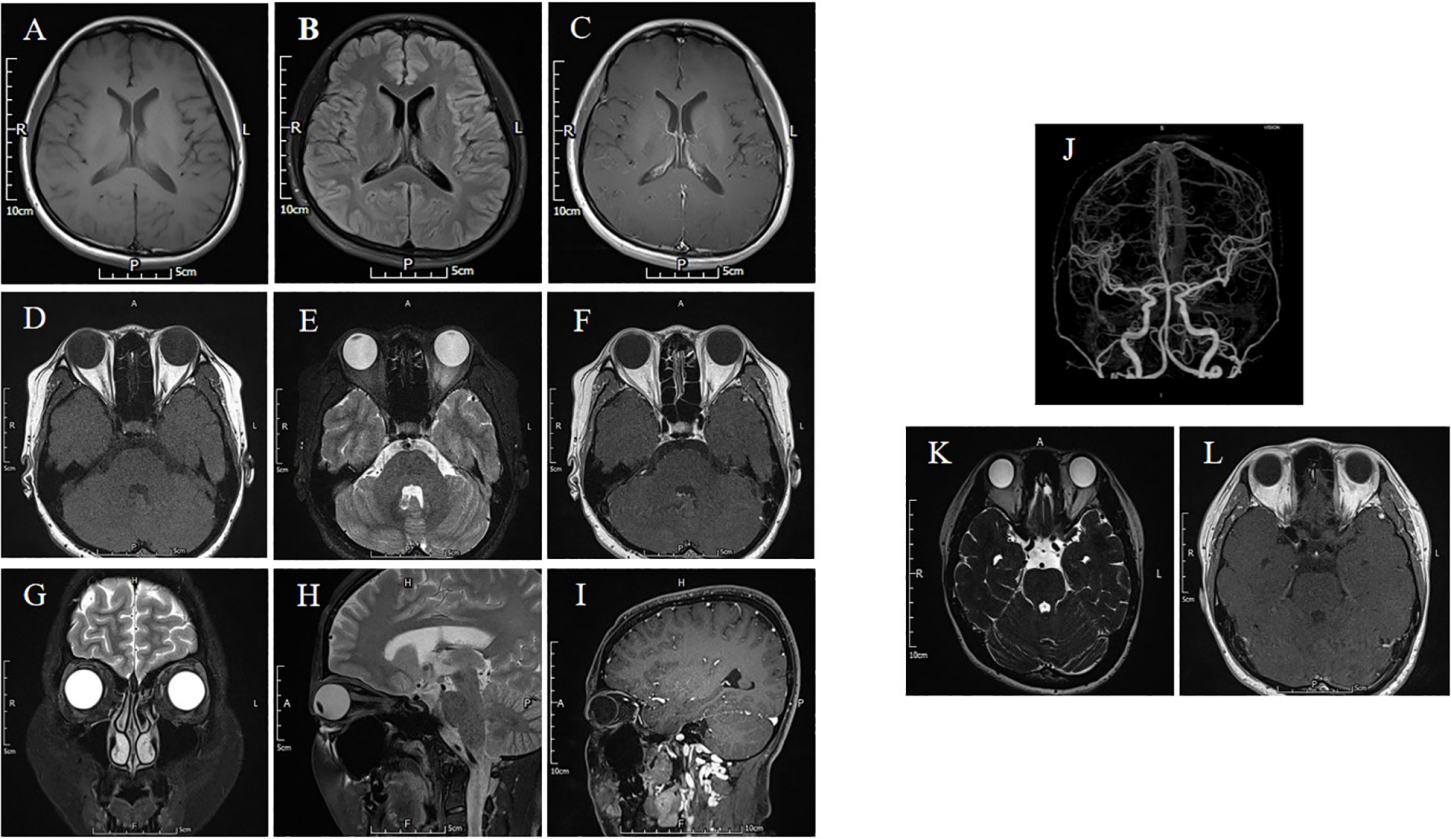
The brain and orbital MRI are normal. (A-C) The T1-weighted, T2 FLAIR and T1-weighted contrast-enhanced sequence of barin. (D-F) The T1-weighted, T2 FLAIR and T1-weighted contrast-enhanced sequence showed no ocular muscle abnormalities. (G) The orbital coronal MRI is normal. (H, I) The T2 FLAIR and T1-weighted contrast-enhanced sequence of orbital sagittal MRI. (J) The CTA of cranial arteries. (K, L) The MRI of oculomotor nerve in T2 Space and T1-weighted contrast-enhanced sequence is normal.
